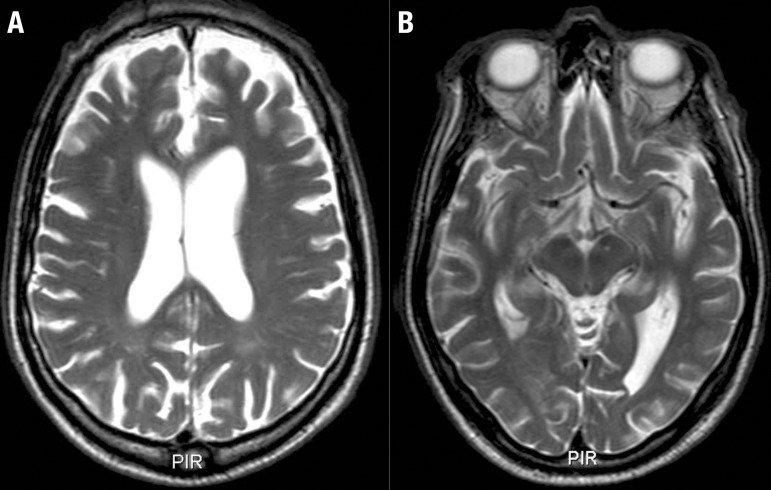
Brain MRI (T2-weighted axial sections) revealed: [A] moderate pre-frontal atrophy, especially in the medial and dorsolateral areas and asymmetric ventricular dilatation (greater in the left hemisphere); [B] asymmetric atrophy (greater in the left hemisphere).
